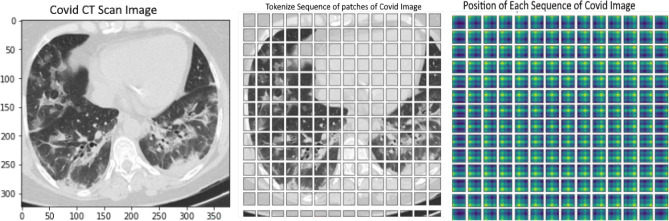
(a) COVID CT scan image, (b) number of patches (c) positional embeddings.
